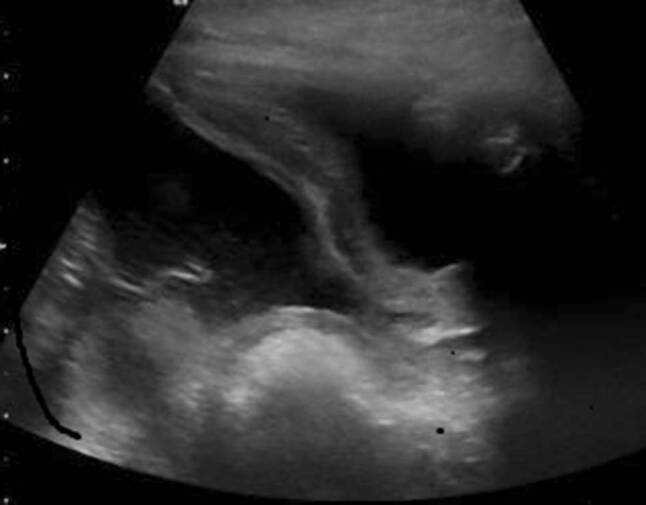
Ultra-sonography showing large intra-abdominal pseudocyst
